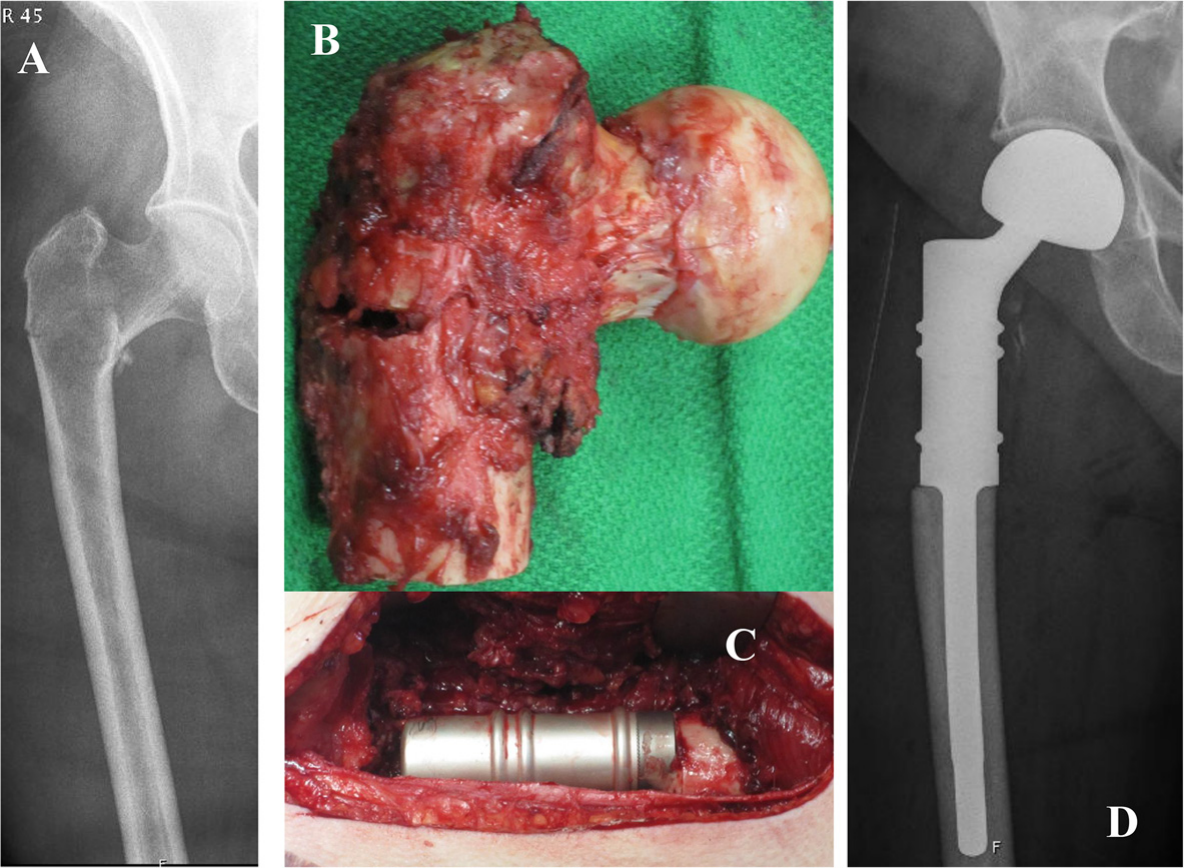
Proximal femoral replacement arthroplasty. A) Preoperative X-ray, a.p. view. B) Completely resected proximal femur including the pathologic fracture, soft-tissues and capsule left in situ. C) Intraoperative view of a proximal femoral replacement after reconstruction of the capsule. D) Postoperative X-ray showing the prosthesis with a bipolar head, a.p. view.
